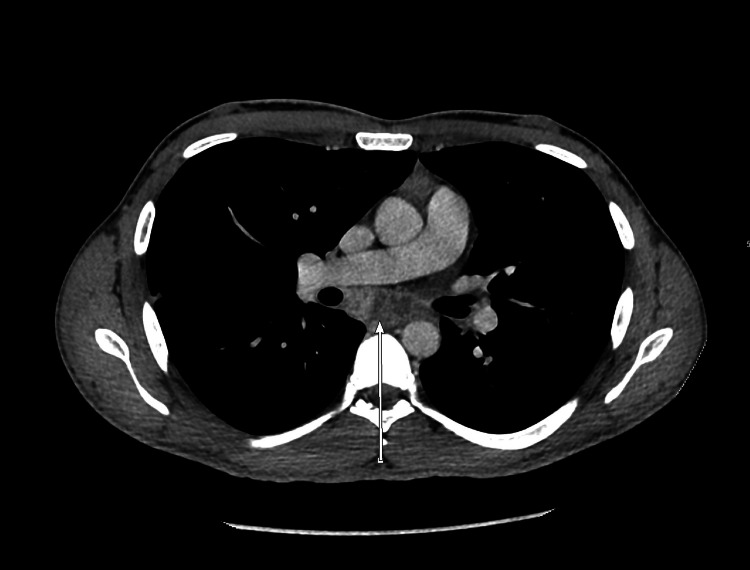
CT scan chest (axial view) at the time of admission The arrow shows the enlarged subcarinal lymph node (29 mm).
